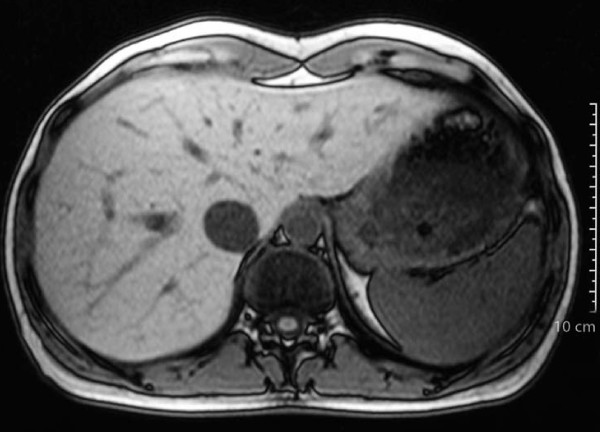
Magnetic resonance imaging scan taken in 2003. The hepatic lesion has disappeared.
